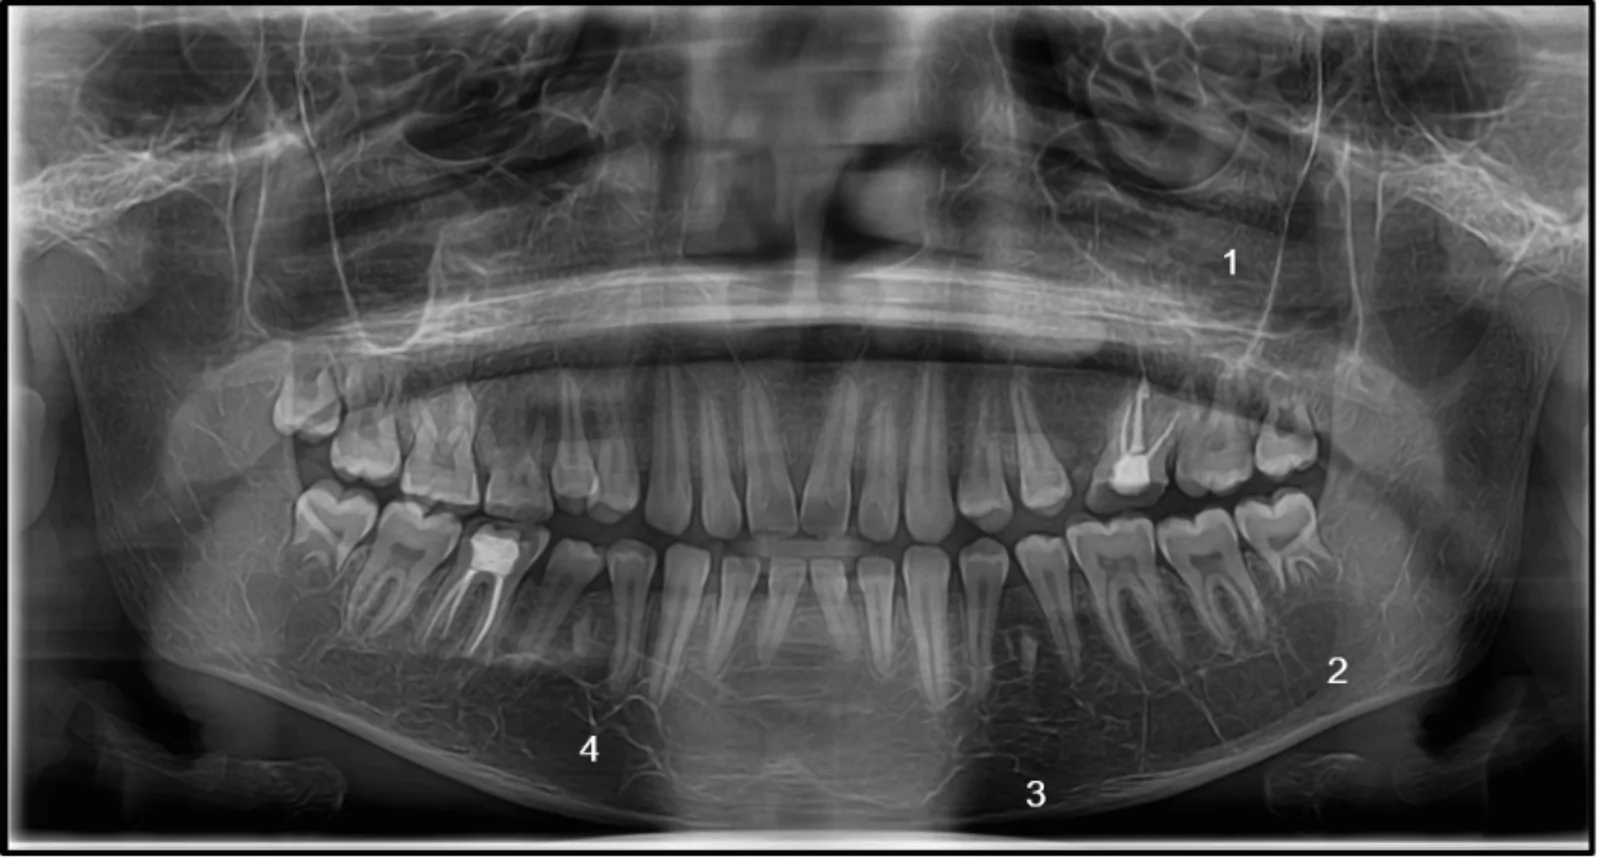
Representative panoramic radiograph of a patient with beta thalassemia major demonstrating characteristic osseous changes. (1) Obliteration of the maxillary sinus, (2) reduced radiographic visibility of the inferior alveolar canal, (3) thinning of the inferior mandibular cortex, and (4) enlargement of the mandibular marrow spaces
